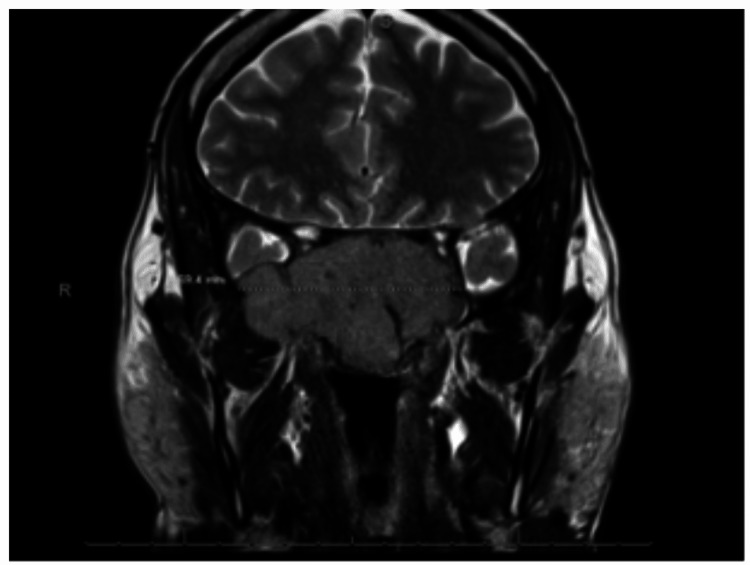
Pituitary magnetic resonance imaging (MRI) MRI depicting a large 7-cm skull base sellar suprasellar mass.
